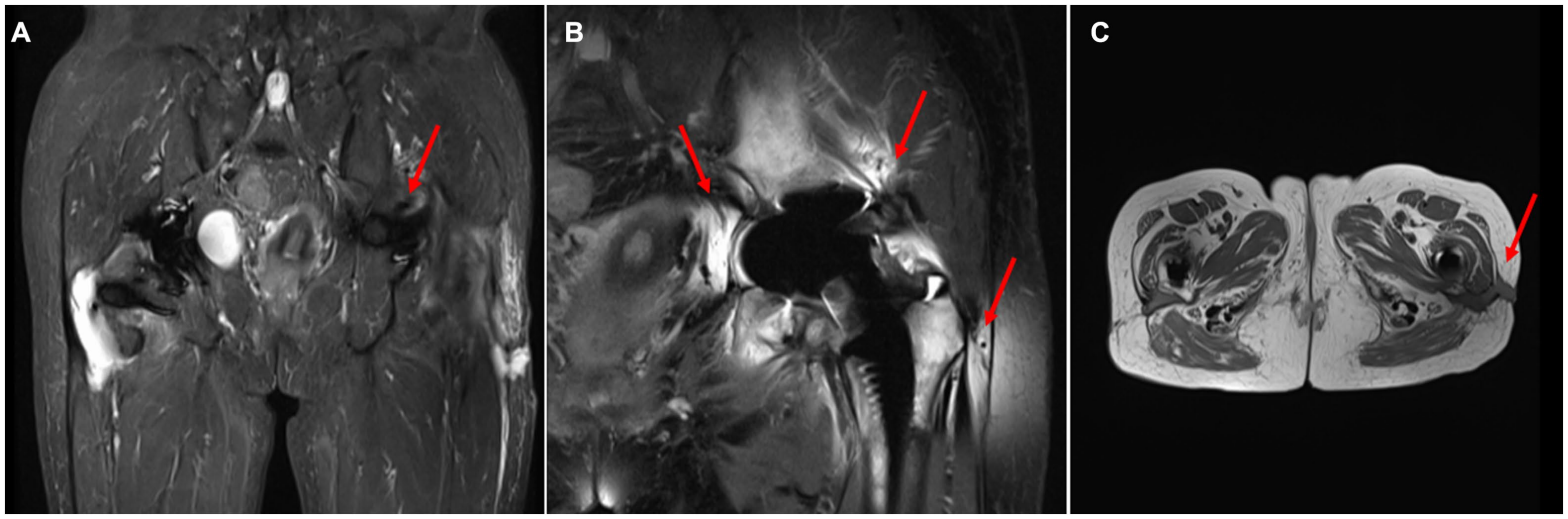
MRI of the hip joint. (A) Localized hyperintensity was found in the skin and fascia of the lateral thigh on the left side(T2). (B) The bone and muscle around the left upper femur were hyperintense(T2). (C) Subcutaneous abscess and fistula formed in the upper part of the left thigh.
